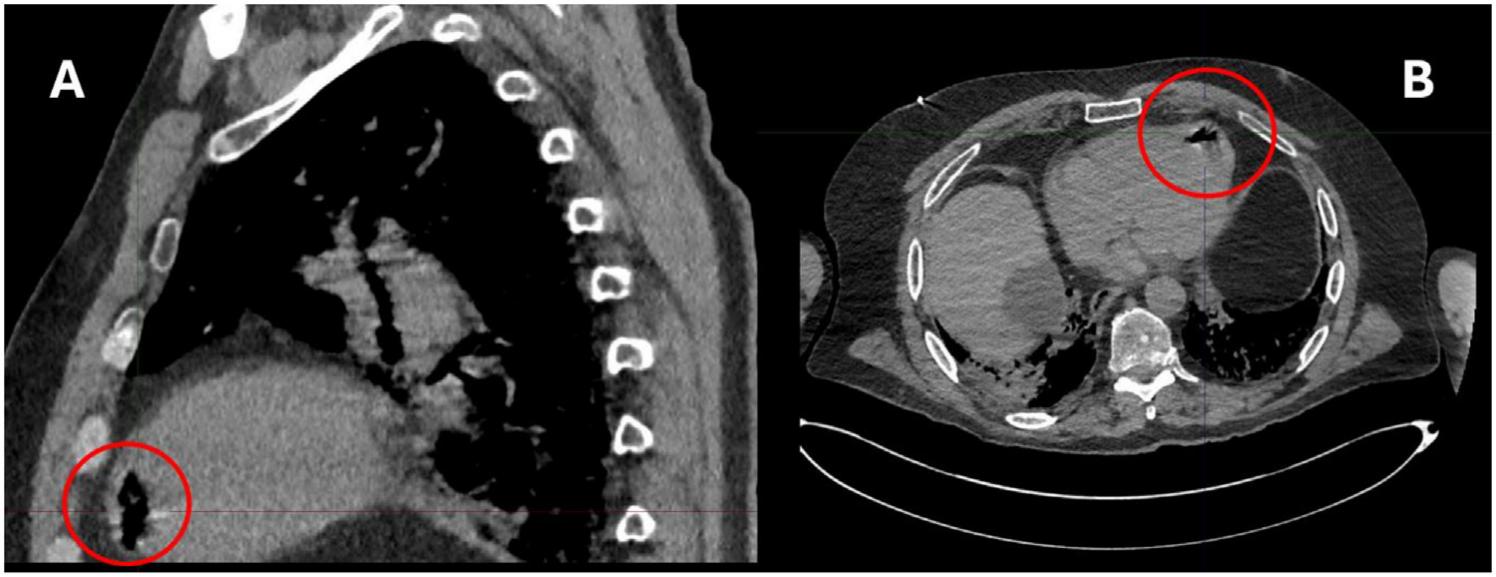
CT scan post-biopsy soft tissue view intraventricular air. (A) sagittal view (B) axial view.
